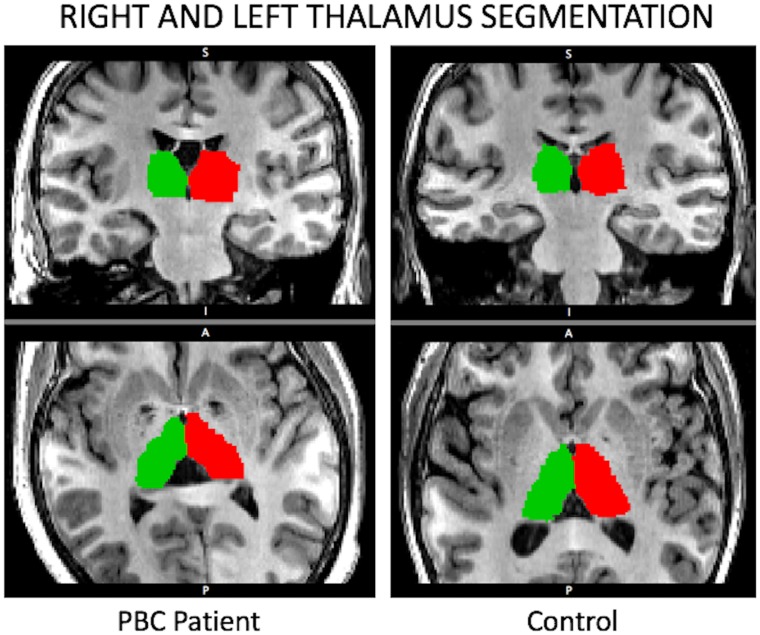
Example segmentations of the right (green) and left (red) thalamus from high-resolution anatomical T1-weighted MRI, for a PBC patient and a control subject.
